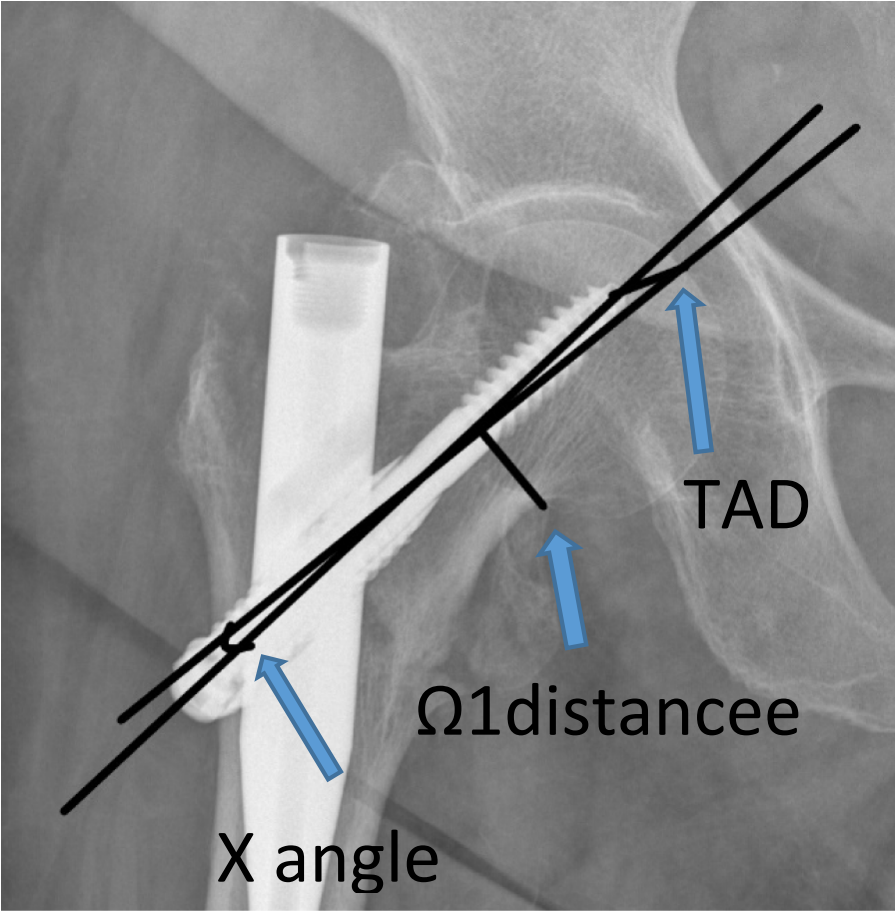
Radiographic measurements obtained from the follow-up radiographs. The diameter of the neck was measured at the point where the roundness of the head ends of the head and the distance of the lag screw from the lower border of the neck and the distance of the anti-rotational nail from the upper border of the neck, for these distances the letter Ω due to its shape, Ω: the diameter of the neck, Ω1: the distance of the lag screw from the lower border of the neck and Ω2: the distance of the anti-rotation nail from the upper border of the neck. The posterior slippage of the lag screw in the first postoperative months was measured as the distance of the lag screw from the lower border of the neck and the TAD give us the position of the nail, i.e. how low and how deep it is located, and the angle X informs about its direction
